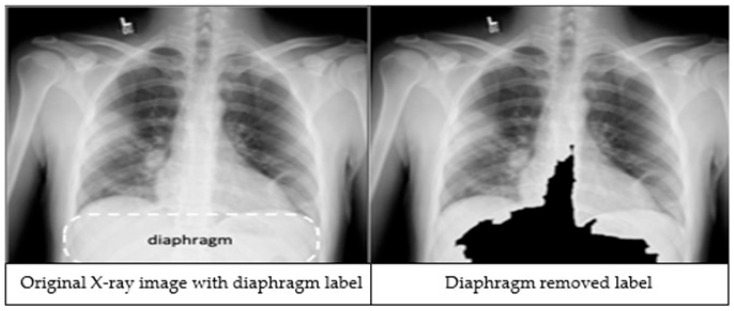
An X-ray image showing the diaphragm region of the image within the dashed lines (left). The image after applying the diaphragm removal technique (right).
